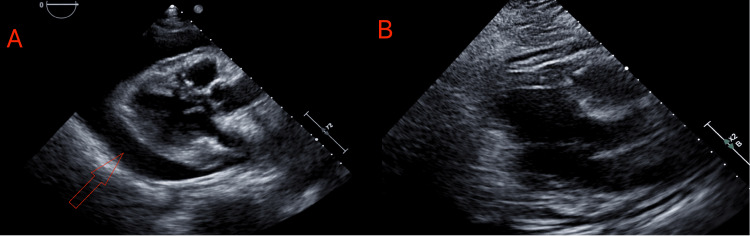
Transthoracic echocardiography before (A) and after (B) pericardiocentesis. (A) A large pericardial effusion before drainage and (B) marked reduction in the effusion after pericardiocentesis.
